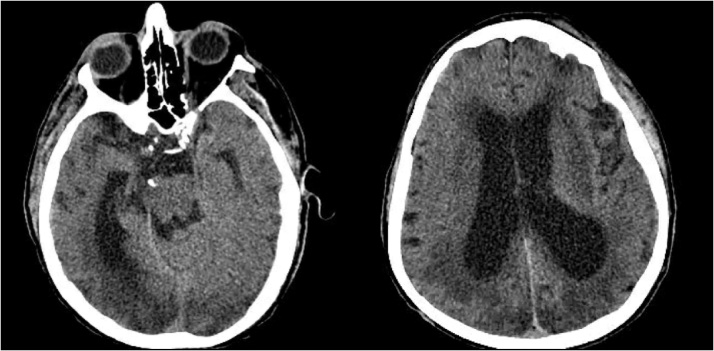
CT Scan of the head showing small linear areas of hyperintensity in the posterior occipito-parietal junction as well as enlarged ventricles suggesting the recurrence of hydrocephalus.
